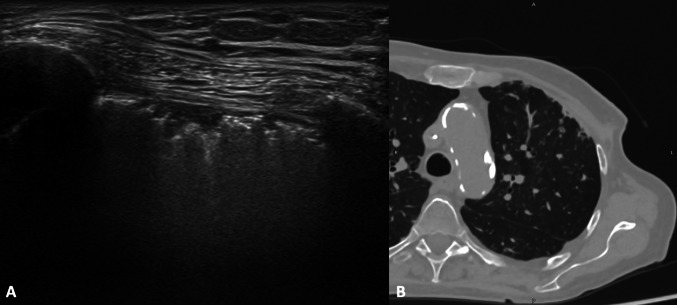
Female patient of 70 years old. B-mode US (A) and CT (B) scans. US demonstrates irregular pleural thickening as a hypoechoic band, superficial to the echogenic pleural-lung interface, suspicious of subpleural fibrosis in patient with mastectomy. CT confirms the diagnosis
